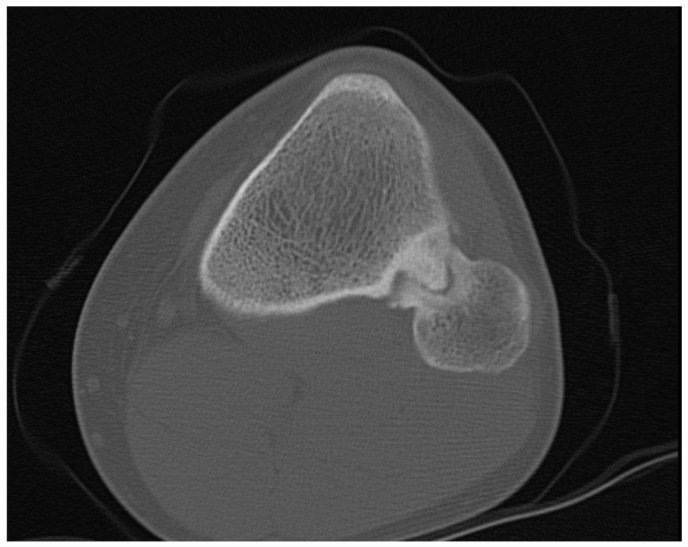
CT scan in horizontal view showing the synostosis of the left side.
